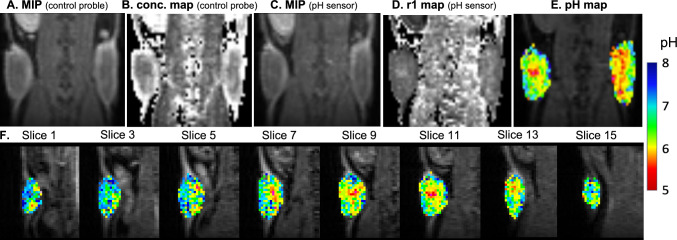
An example of RpH-MRI of Fe-tCDTA (1st injection as control) and pH-sensor (2nd injection) mapping the tumor pHs. A T1-weighted image with maximum intensity projection (MIP) of the first injection (control probe); B concentration (conc.) map of the first injection (control probe); C T1-weighted image with MIP of the second injection (pH sensor); D T1 relaxivity (r1) map of the second injection (pH sensor); E pH map; F demonstrates pH maps of various slices of the left tumor. All shown images are in coronal orientation and dose is 0.25 mmol/kg
